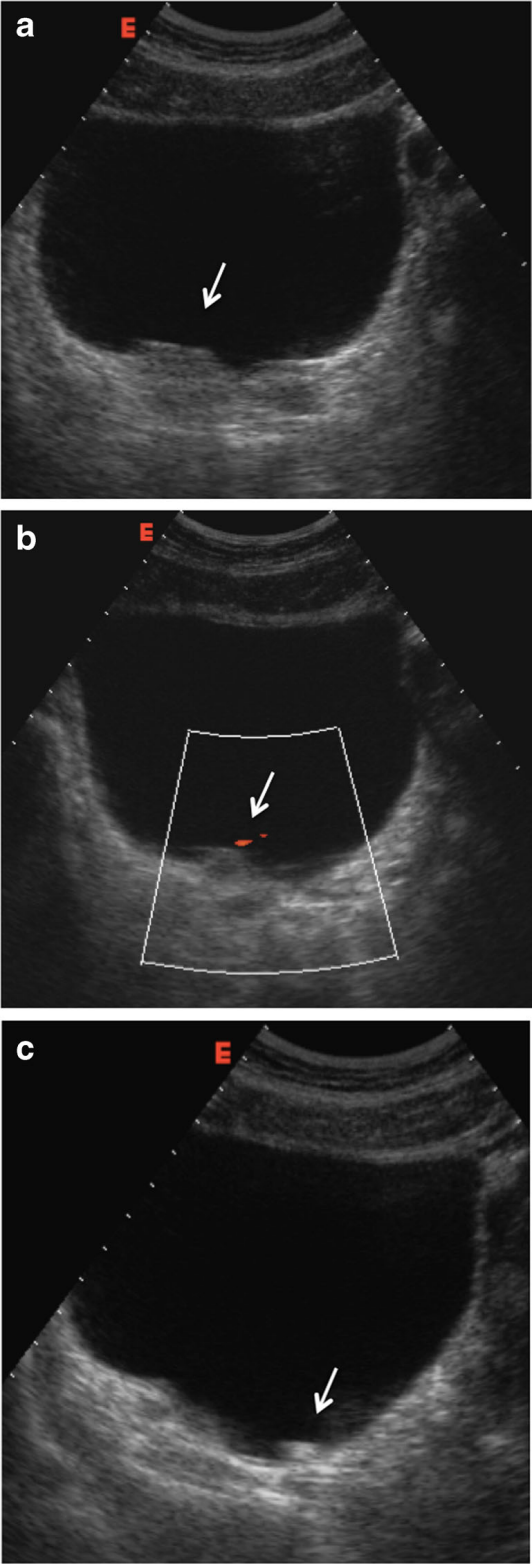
Patient with right renal colic. a US image show an oedematous UVJ (*arrow*). b Colour Doppler shows the presence of a small ureteral jet confirming the patency of the ureter (*arrow*). c A small stone that moves according to patient decubitus is identified at the urinary bladder (*arrow*)
